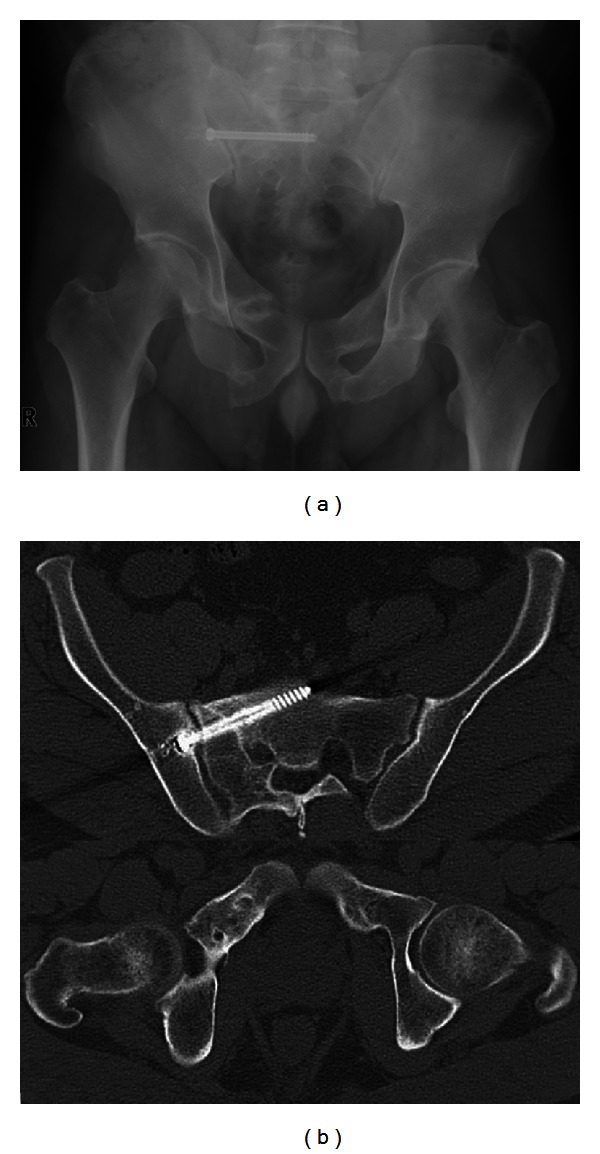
Anteroposterior radiograph (a) and (b) CT images taken 9 months after operation, which demonstrate the healing of nonunion of the right sacrum bone and bilateral superior pubic rami.
